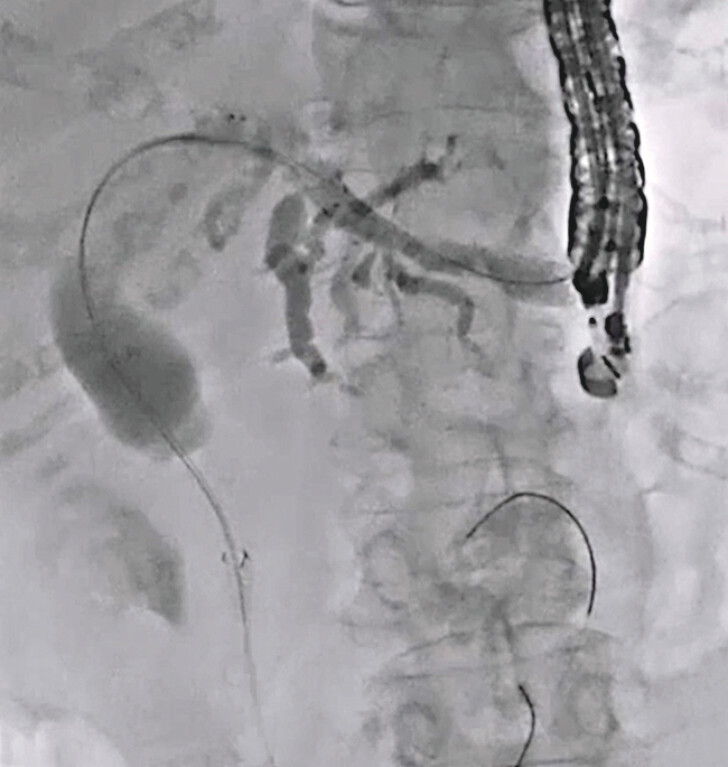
Endoscopic ultrasound-guided hepaticogastrostomy is performed.
